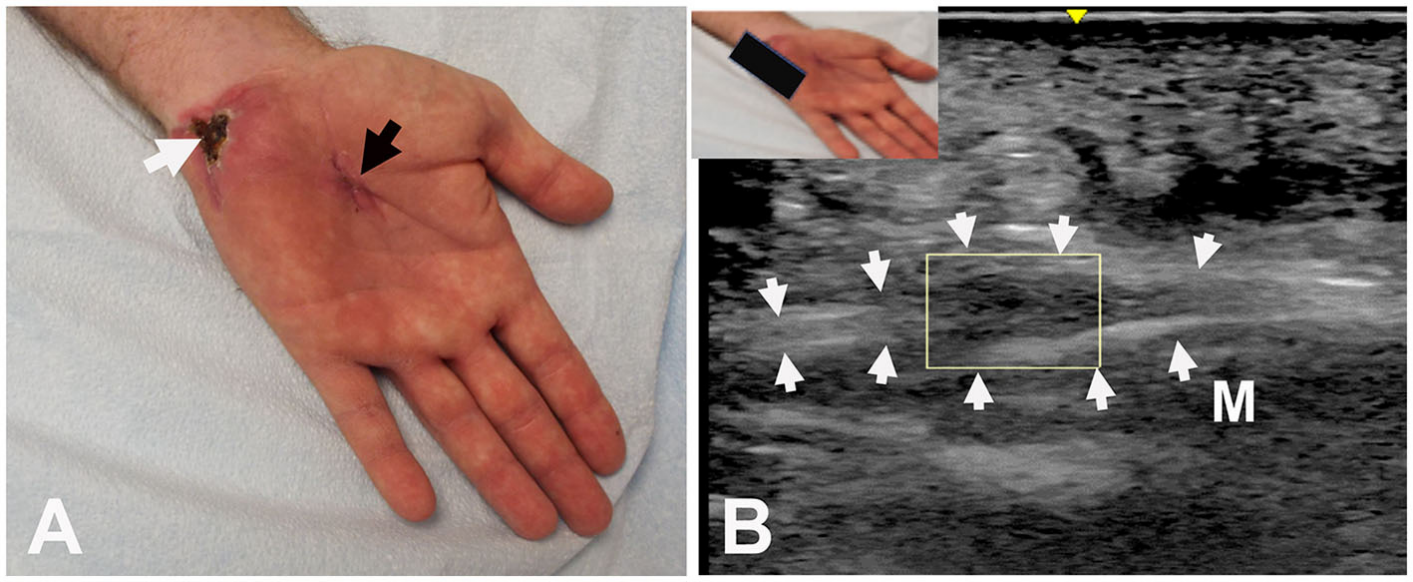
Patient #9: (A) Entry in palm (black arrow) and exit at base of hypothenar eminence (white arrow). (B) Ultrasound: Long axis view shows hypoechoic enlargement of the ulnar nerve (neuroma in continuity) (box). Arrows outline the ulnar nerve. M: Abductor digiti minimi muscle.
